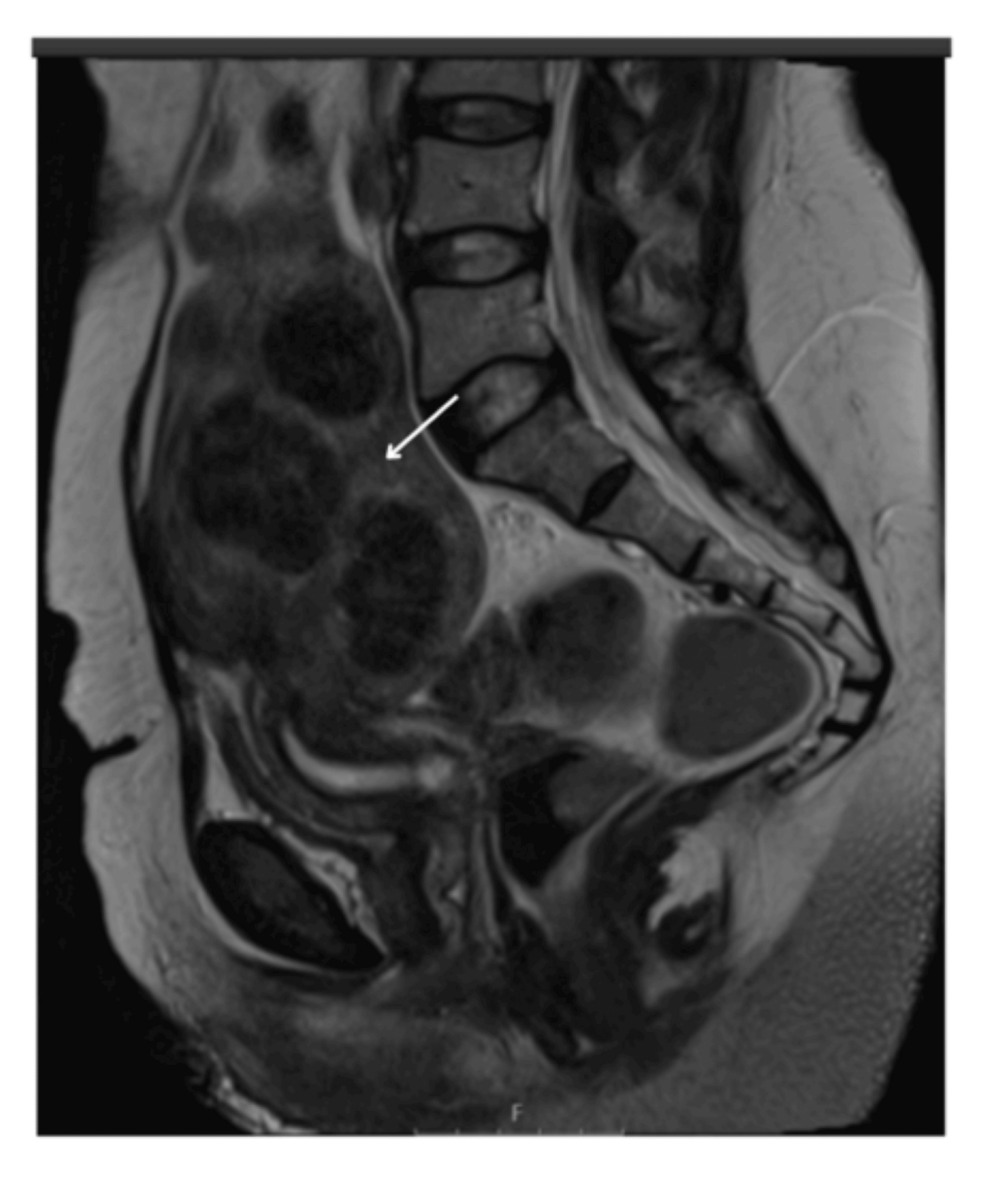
Sagittal T2 weighted large field of view MRI image of the pelvis showing fibroid uterus (arrow), pre-embolisation
